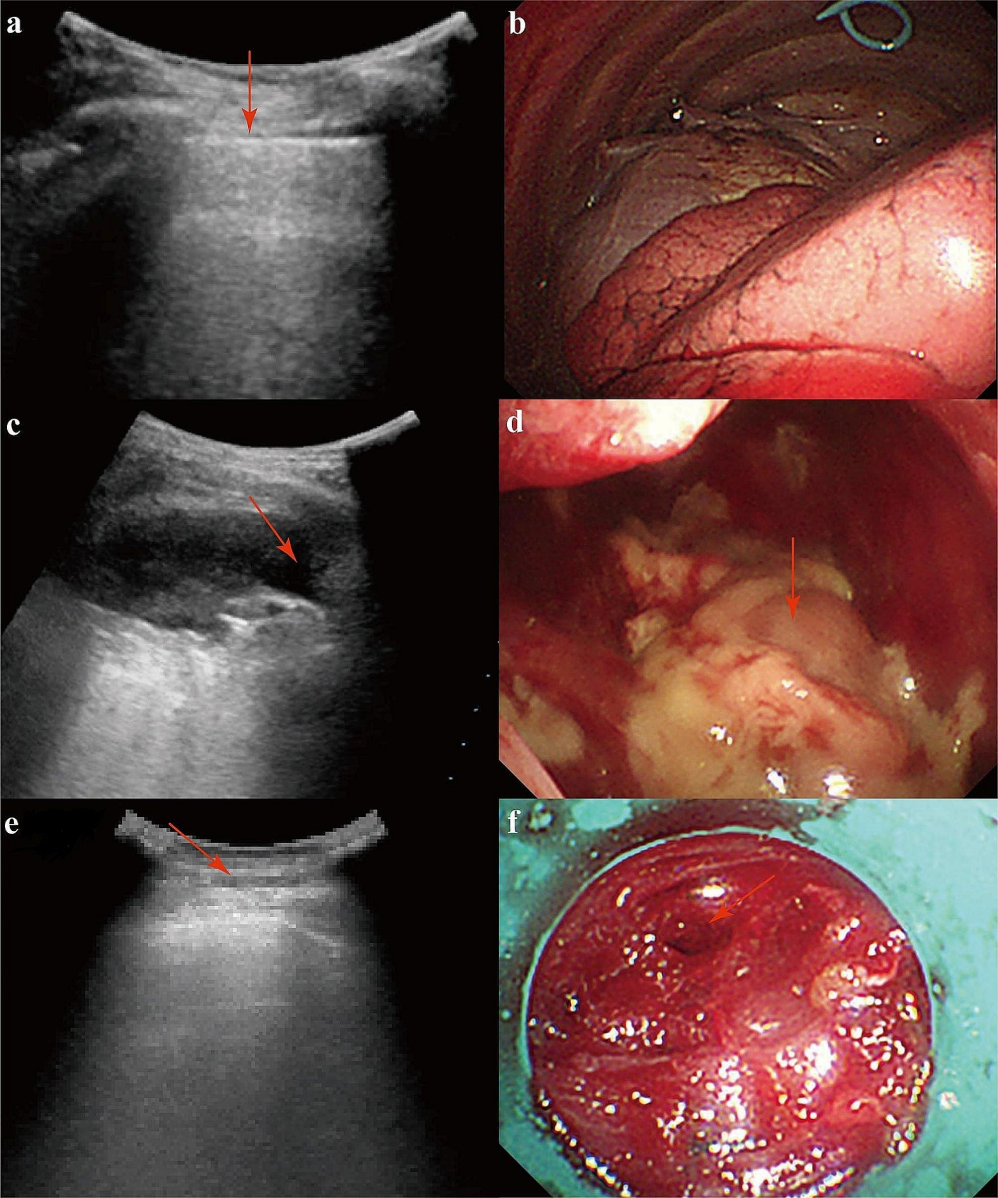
Ultrasound and thoracoscopic presentation of typical patients. (a) Ultrasound presentation in a patient with a pleural sliding sign (red arrow). (b) Thoracoscopic manifestation of the patient is fully exposed and without pleural adhesions. (c) Ultrasound presentation in a patient with a fluid sonolucent area (red arrow). (d) Thoracoscopic manifestation of the patient with encapsulated empyema (red arrow). (e) Ultrasound presentation in a patient showed thickened pleura (red arrow) without pleural sliding sign. (f) Thoracoscopic manifestation of the patient with severe adhesions in the pleural cavity. There was visible injury to the lung (red arrow)
